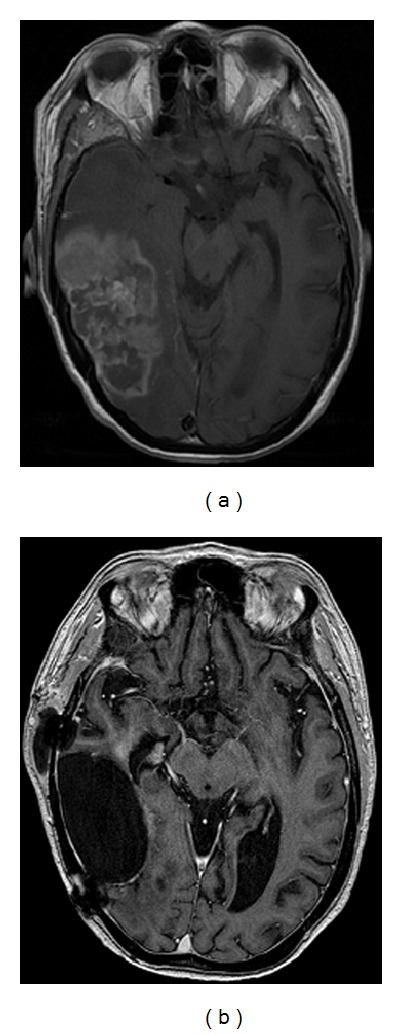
(a) Axial contrast-enhanced MR image of the cerebrum showing a 3.8 × 7.8 cm tumor infiltrating the parietooccipital region of the right hemisphere. The lesion is with surrounding edema and compressing the right lateral ventricle and the right side of the mesencephalon. (b) Four weeks after start of treatment, a resection cavity with a modest enhancement against the calvaria is seen. The centre line is in normal position, and the content of the cavity is isodense with the cerebrospinal fluid.
